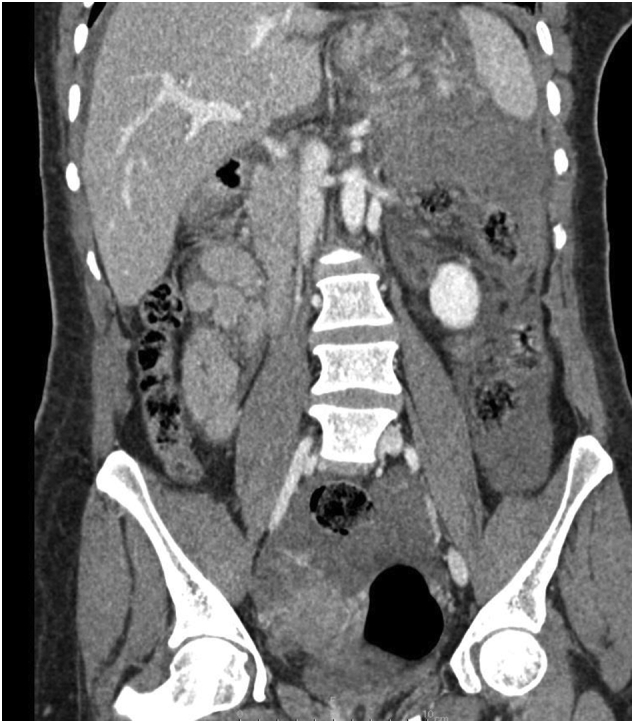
Shows a coronal reconstruction CT scan in the venous phase showing extensive hemoperitoneum.
